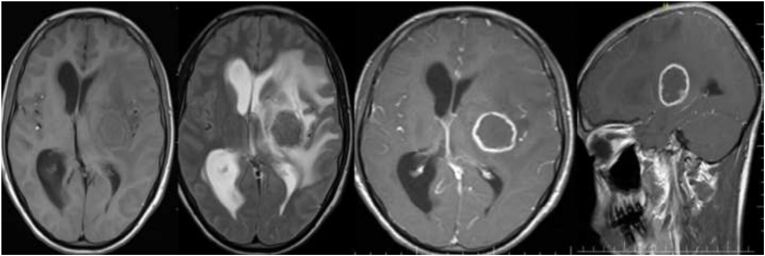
caseating tuberculomas A (T1W axial) appears isointense with a hyperintense rim. B (FLAIR axial) hypointense lesions in the left parietal lobe with perilesional edema, which in post-contrast C (T1W axial) and D (T1W sagittal) show ring enhancement of the conglomerate tuberculomas.
